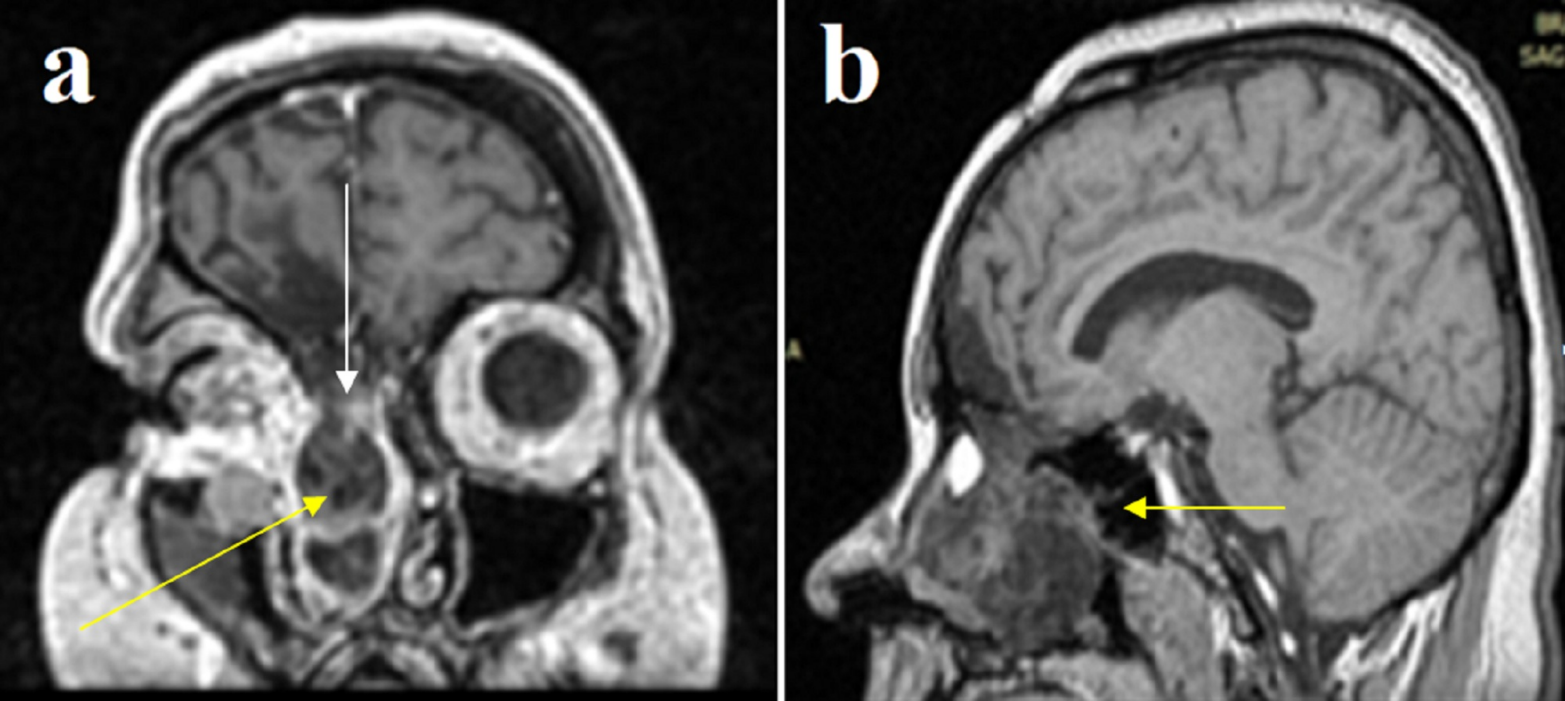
Preoperative MRI brain scan (coronal [a] and sagittal [b] view) demonstrating a large meningoencephalocele extending through a frontal bone gap on the right side (yellow arrows) and a cribriform plate defect with complete opacification of the frontal and anterior ethmoid sinuses (white arrow).
